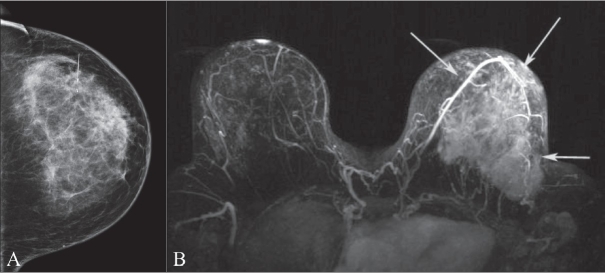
Craniocaudal mammographic projection (A) and MIP DCE-MRI image (B). This 42-year-old female was referred for DCE-MRI to determine the extent of disease after a new diagnosis of invasive ductal carcinoma. Note how much better MRI demonstrates disease (arrows in B) than the mammogram (arrow in A)
